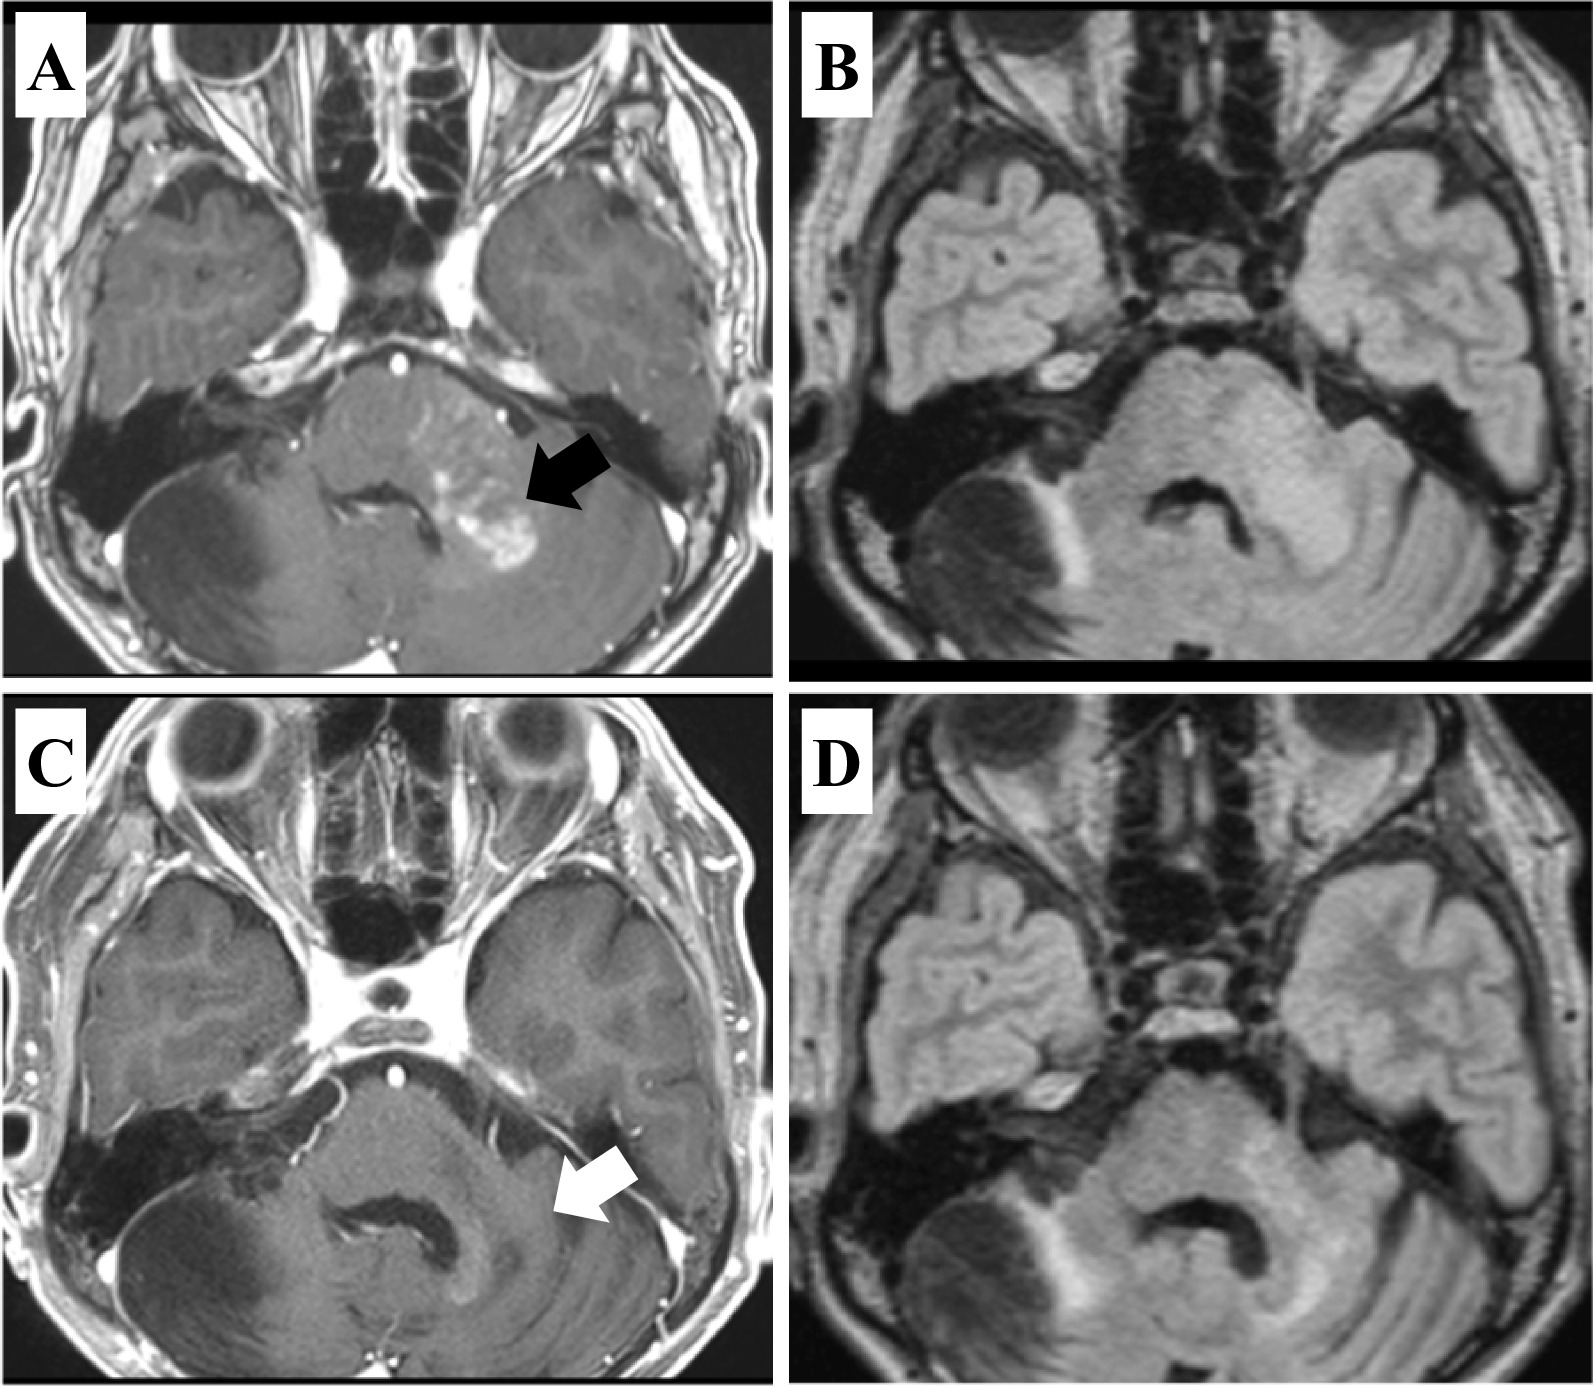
Representative patient treated with reirradiation, temozolomide, and bevacizumab (ReRT/TMZ/Bev) showing a favorable response (Case 11). A Preoperative T1-weighted magnetic resonance image with gadolinium enhancement and B fluid-attenuated inversion recovery (FLAIR) image showing an enhanced tumor in the left cerebellum (black arrow). C T1-weighted magnetic resonance image with gadolinium enhancement and D FLAIR image obtained 9.8 months after ReRT/TMZ/Bev treatment showing a favorable response (white arrow)
